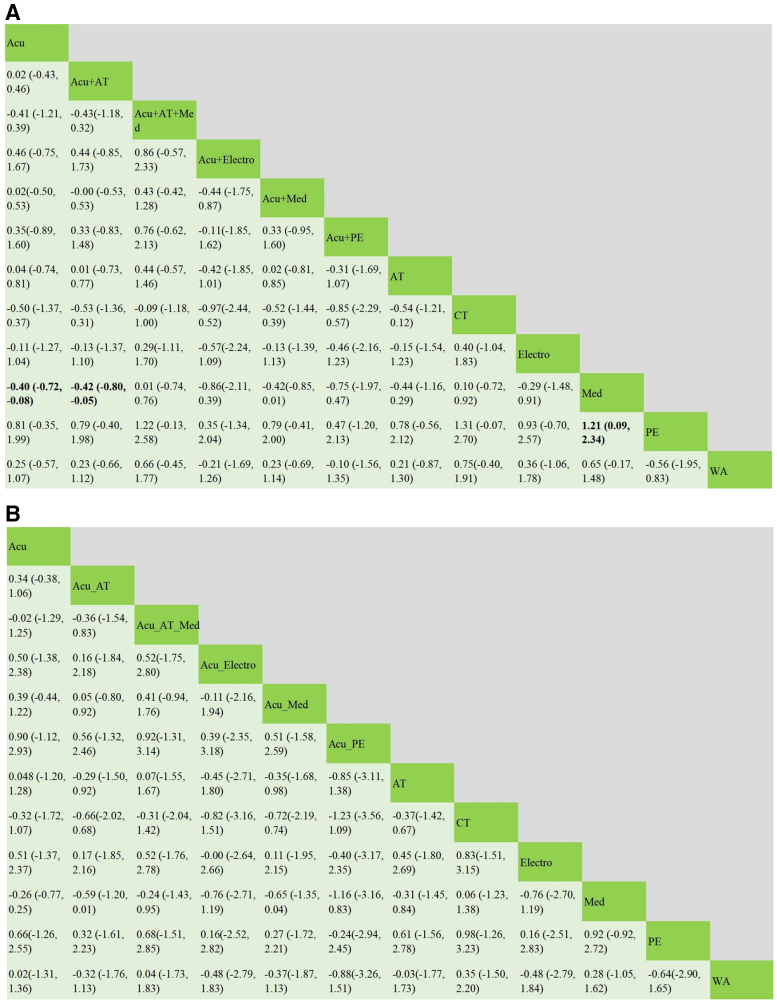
Network meta-analysis results for sleep efficiency and subjective sleep quality. (A) Subjective sleep quality and (B) sleep efficiency. Acu = acupuncture, AT = auriculotherapy, CT = conventional treatment; Electro = electroacupuncture, Med = western medicine, PE = point embedding, WA = warm acupuncture.
